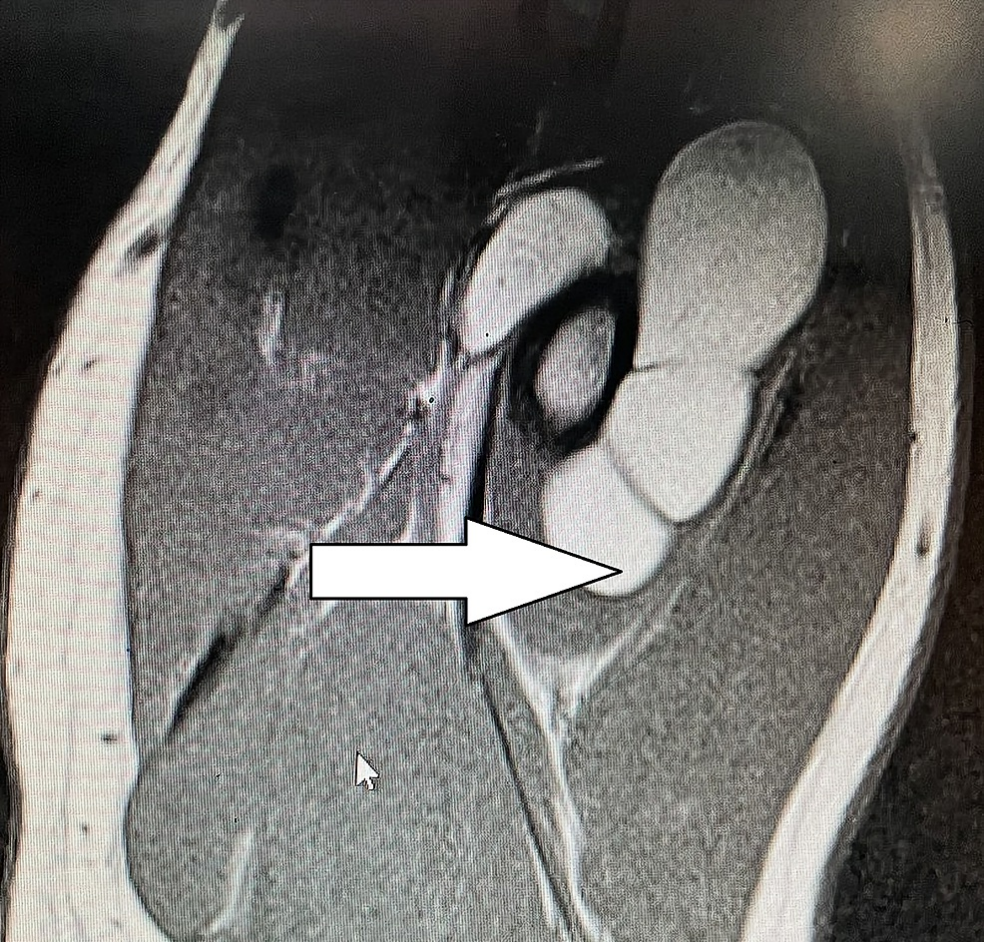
MRI showing the extent of the tumor (arrow) MRI: magnetic resonance imaging
